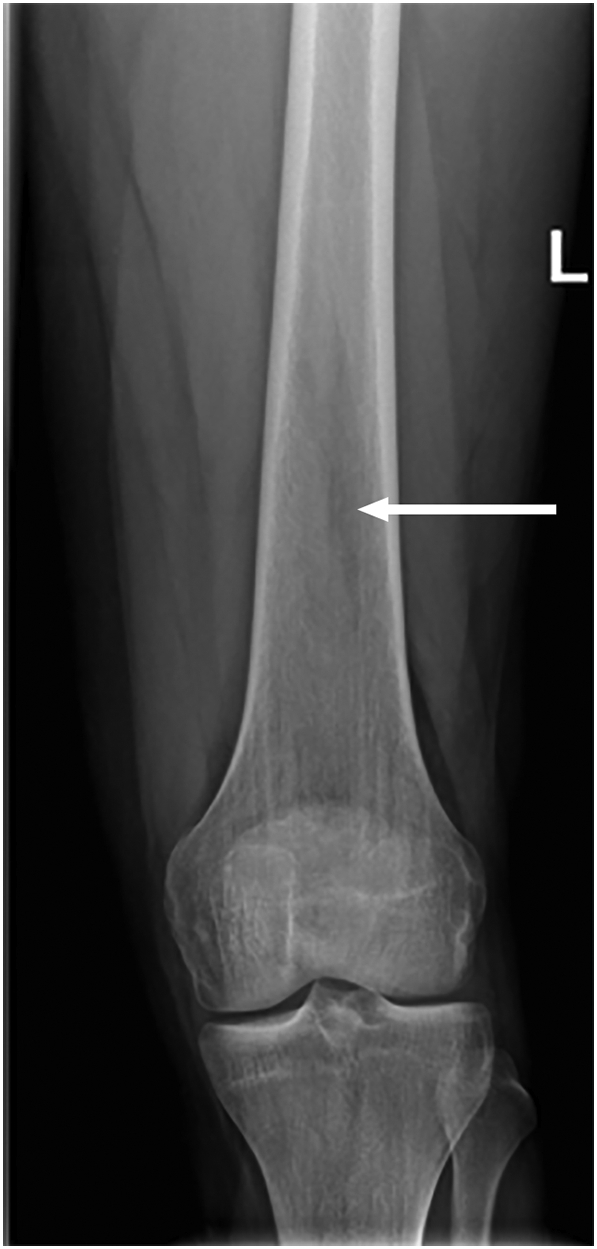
Conventional X-ray of the left distal femur, showing intramedullary sheath-like lucencies in the distal femoral diaphysis.
